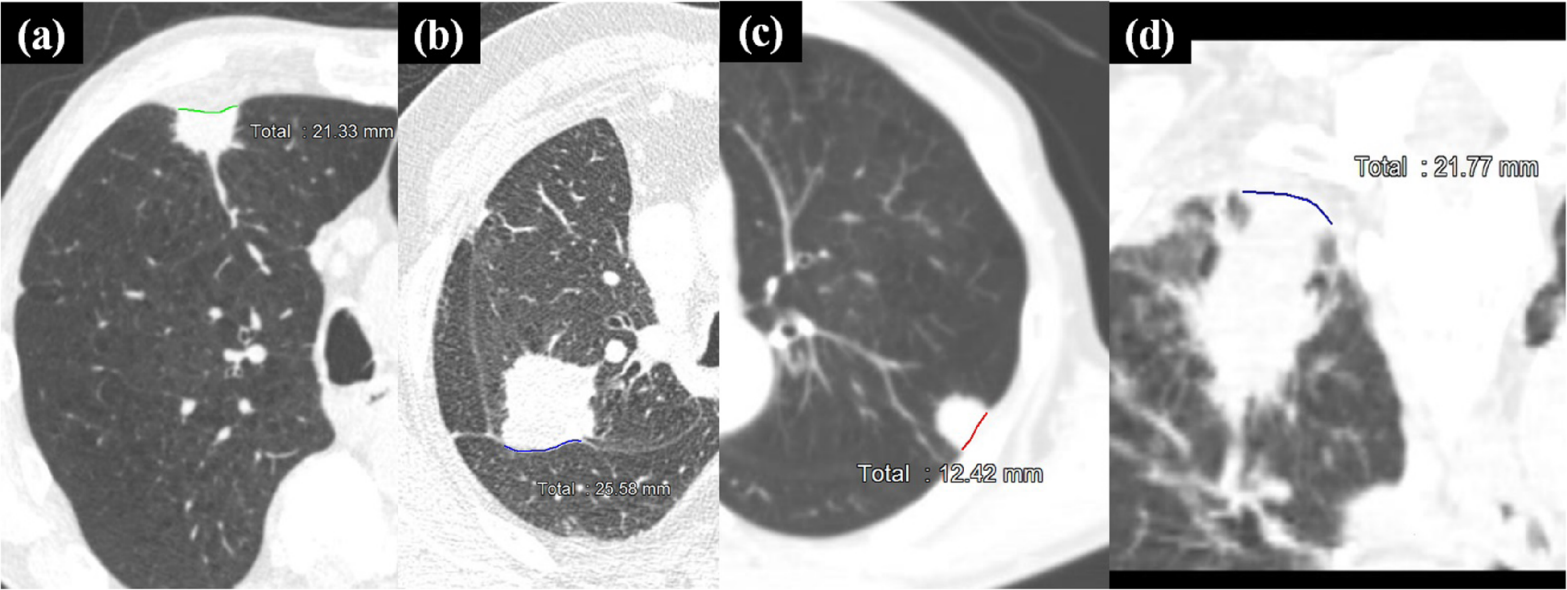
Length of tumor attachment to the pleura were measured at the lung window. Measurements of tumor attachment to the visceral pleura (a), tumor attachment to the interlobar pleura (b), tumor attachment to the pleura in non-high-resolution CT (c), tumor attachment to the pleura at a coronal section (d).
